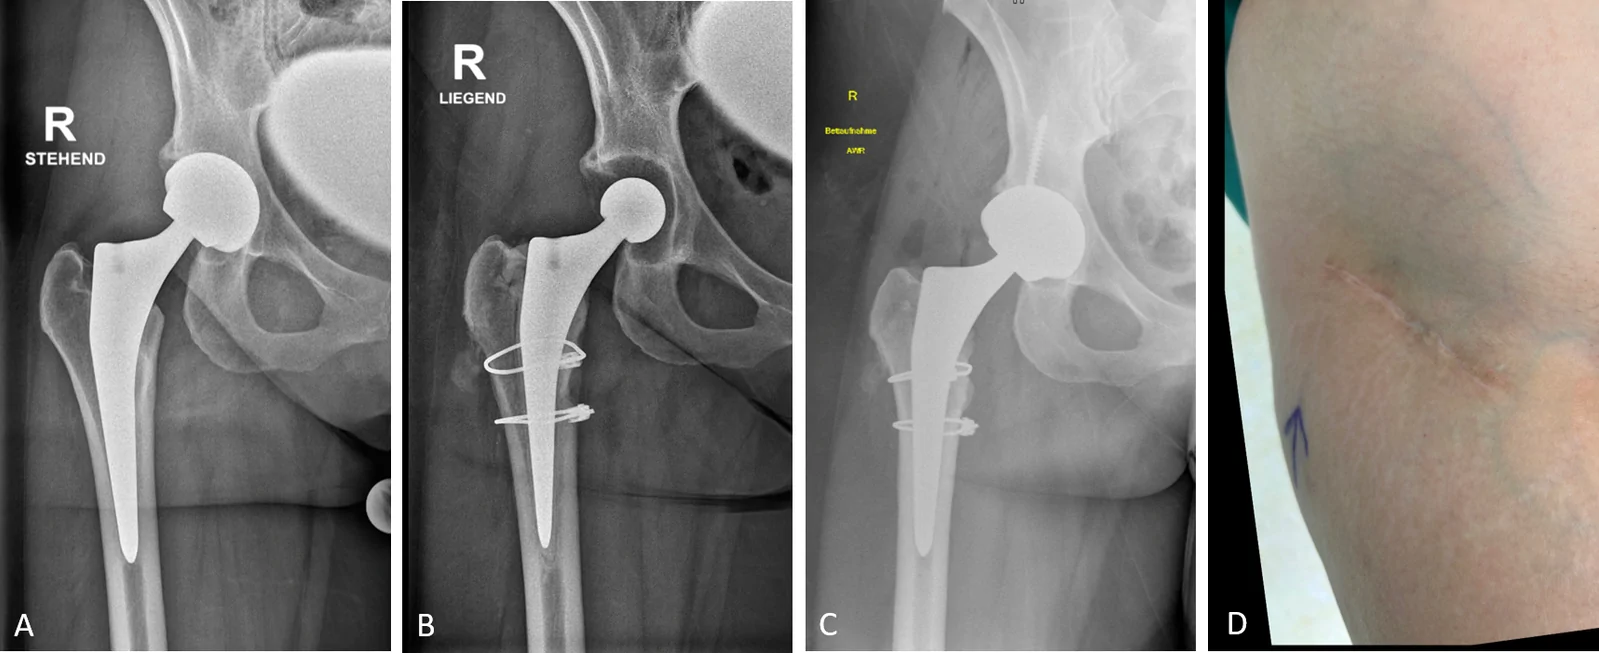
(A) shows a postoperative anteroposterior (AP) X-ray of a primary total hip arthroplasty (THA) using the direct anterior approach (DAA) with a bikini incision, (B) shows a postoperative ap radiograph with a custom-made spacer and two cable wires after femoral component removal and femoral osteotomy using the DAA, (C) shows a postoperative ap radiograph with a successful second-stage reimplantation. (D) Scar after 3
